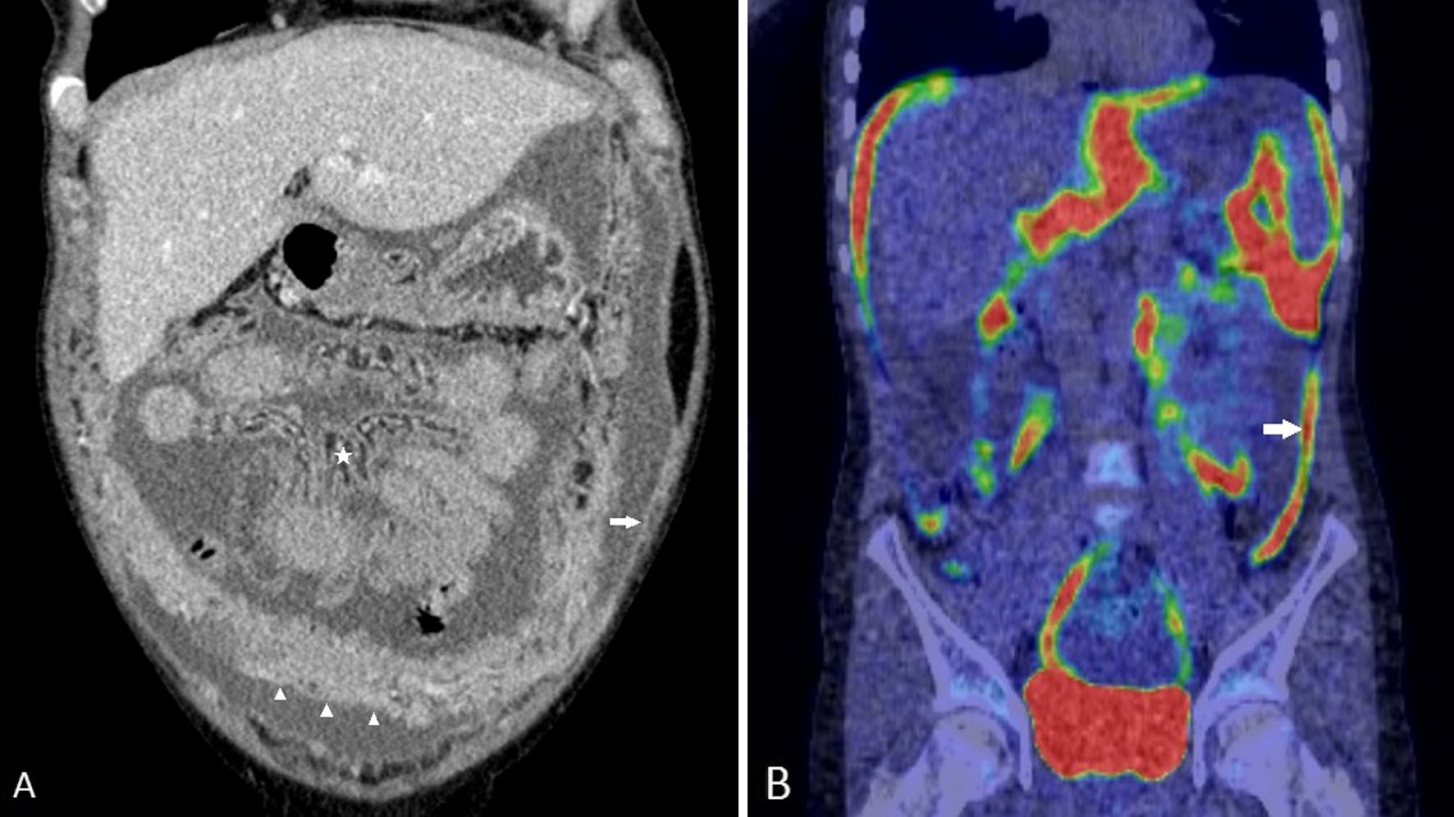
a, b 47-year-old female with epithelioid type of malignant peritoneal mesothelioma. a Coronal CT image demonstrates diffuse plaque-like peritoneal thickening (white arrow), omental caking (arrow heads), ascites and tethered mesentery (*). b Coronal PET-CT shows FDG-avid plaque-like thickening (arrow) of the peritoneum
